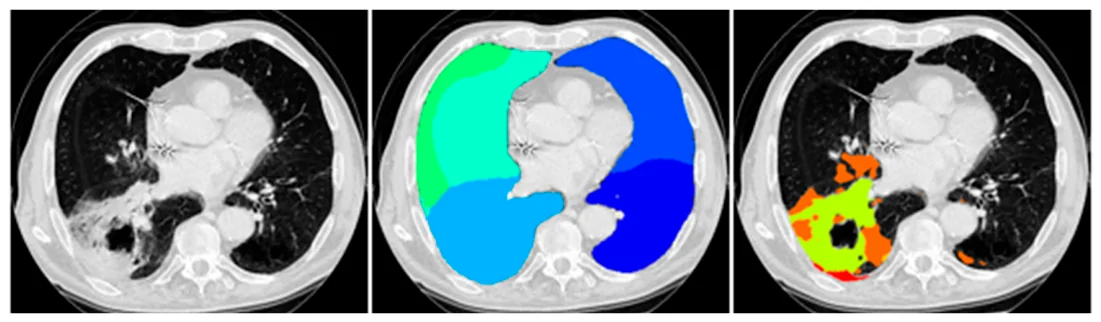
Fully automated evaluation of a chest CT scan by an AI-based software tool (ADVANCE chest CT, contextflow GmbH, Vienna, Austria). (left) CT image without any software overlays, (middle) automated segmentation of the lung lobes (light green, right upper lobe; turquoise, middle lobe; light blue, right lower lobe; blue, lingula; dark blue, left lower lobe), (right) AI-generated detection of circular consolidations in the right lower lobe, marked in yellow, as well as GGO in both lower lobes and in the middle lobe, marked in orange. CT, computed tomography; AI, artificial intelligence; GGO, ground-glass opacities.
